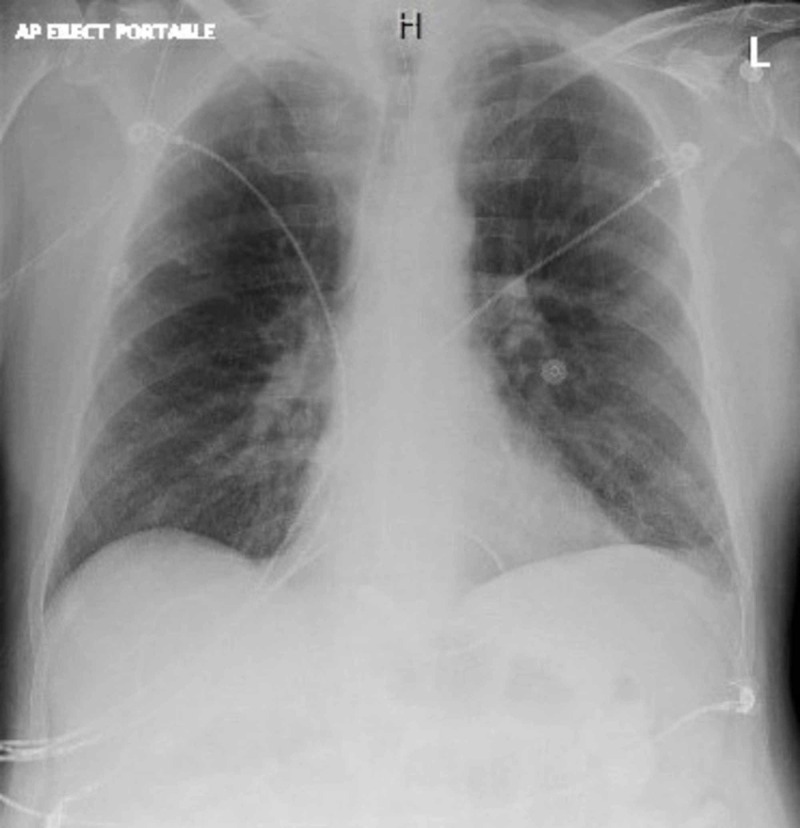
Portable chest X-ray negative for free air.
